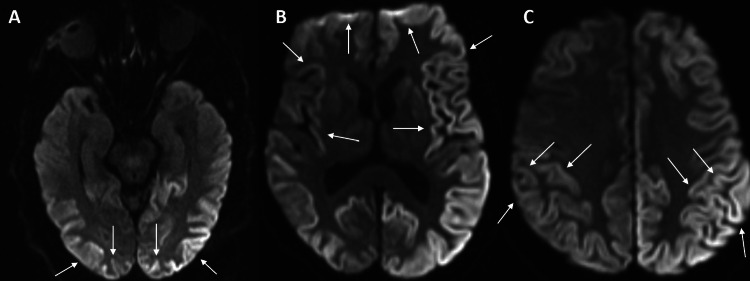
MRI (axial view) of Case 3 with cortical hyperintensity in DWI sequence The arrows point to the cortical ribboning of the bilateral (A) occipital, (B) frontal, temporal, and (C) parietal lobes. DWI: diffusion-weighted imaging
